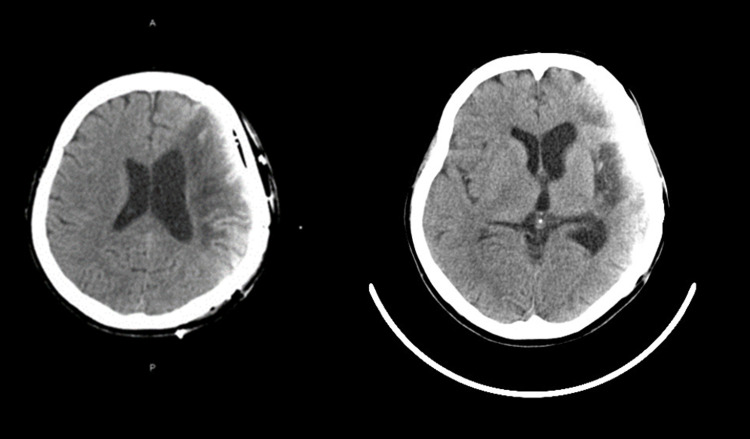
Preoperative head CT. Plain head CT showed old vascular lesions over the left hemisphere, which were associated with right spastic paresis. CT: computed tomography
